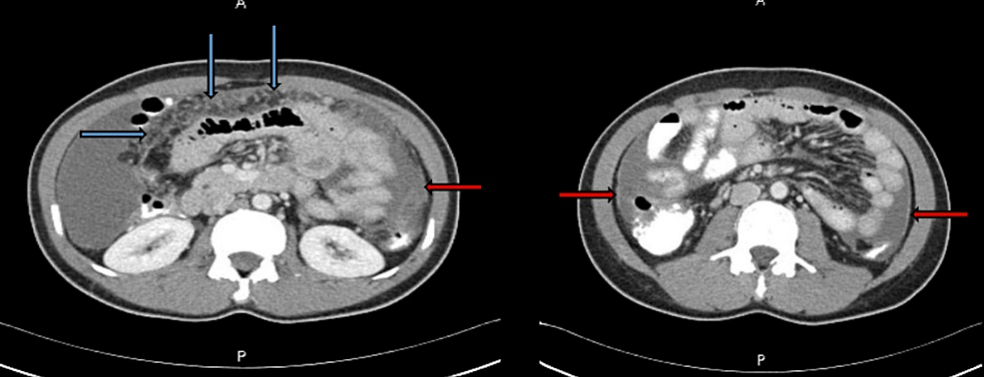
Abdominal CT scan showing diffuse omental thickening and cake (blue arrows) and peritoneal thickening (red arrows).
